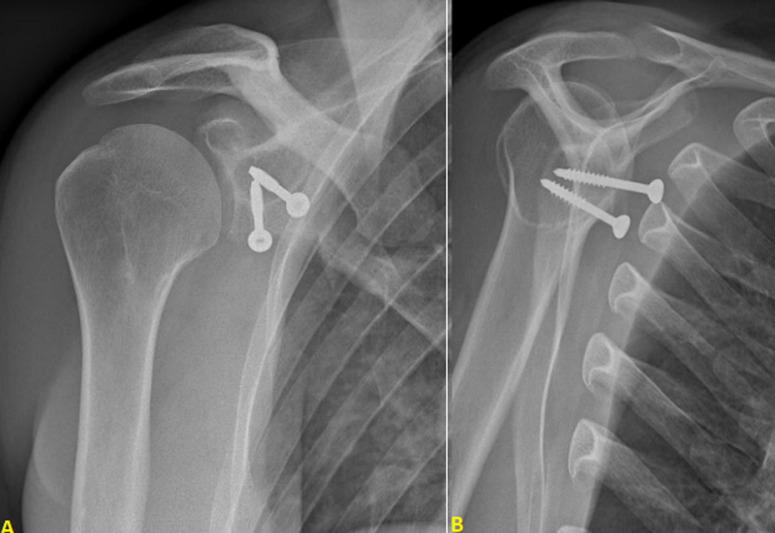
(A,B) frontal and lateral X-rays of right shoulder showing a geode in scapular neck
